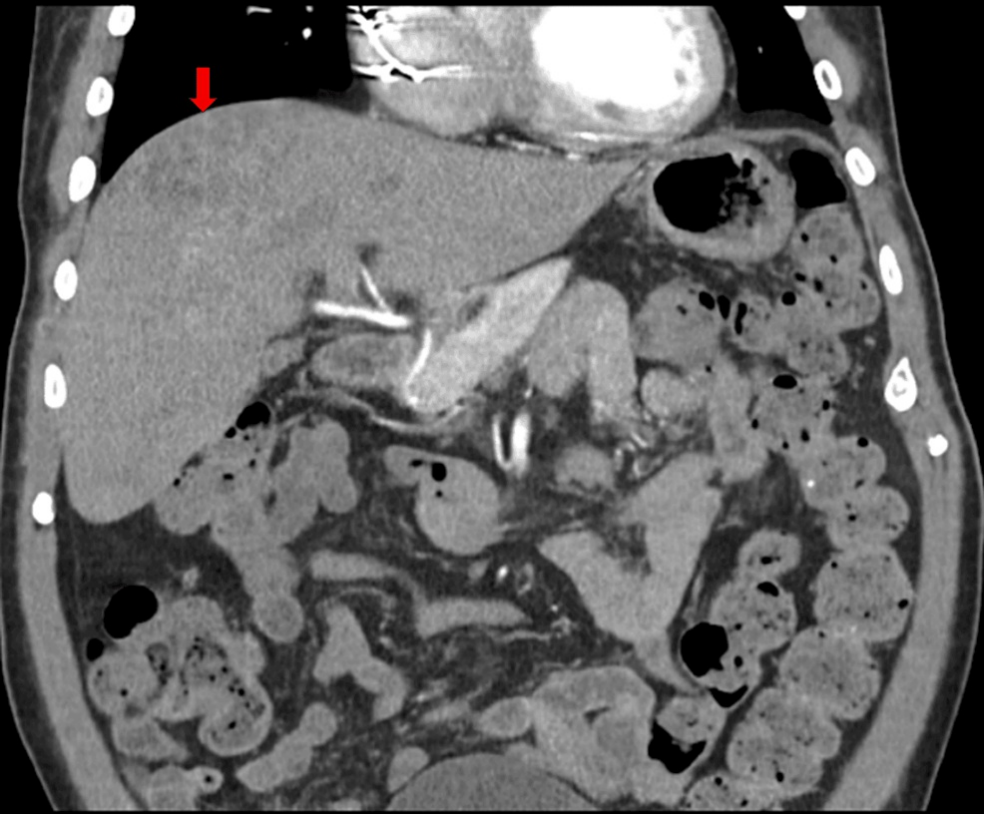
Contrast-enhanced liver CT Wedge-shaped heterogeneous areas of low attenuation in segment VIII of the liver, likely the sequela of prior infection but no evidence of abscess.
